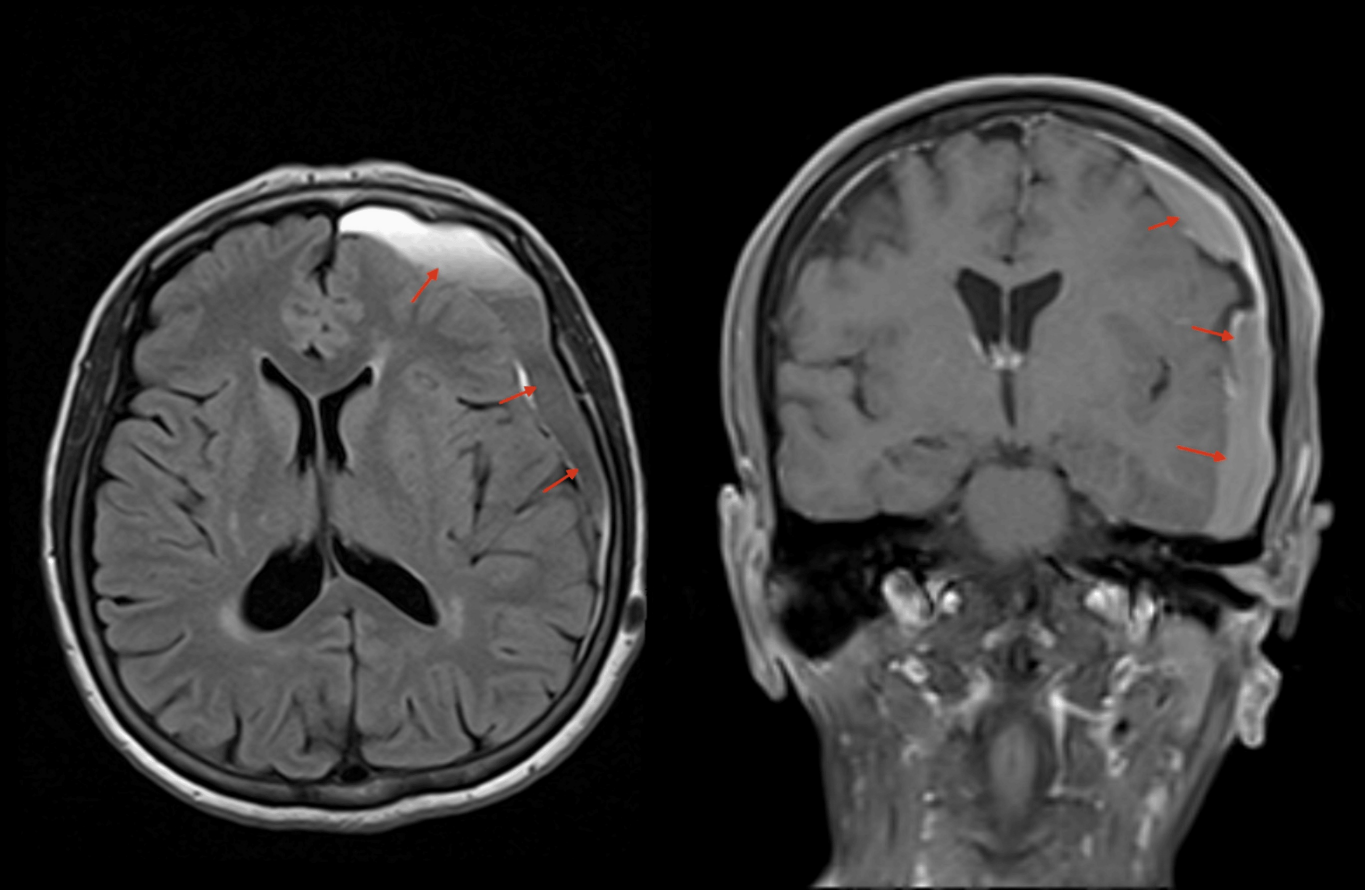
Brain MRI post-MMA embolization. Follow-up axial and coronal brain MRI demonstrates bilateral cerebral convexity subdural hematomas with interval stability in terms of maximum thickness and midline shift. MMA: Middle meningeal artery.
